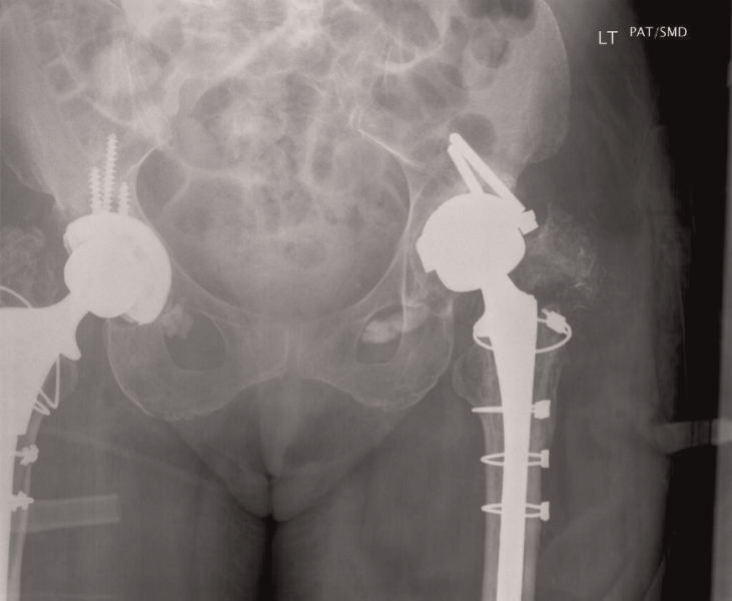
Radiograph at 8^th^ month postoperative period showing broken acetabular screws.
